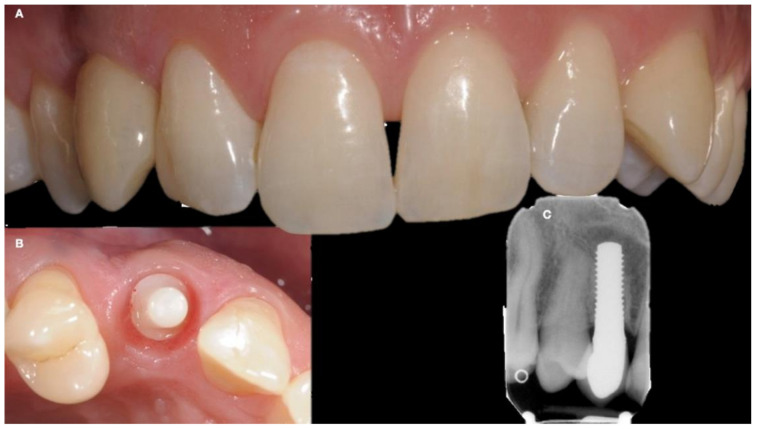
Definitive crown cementation (A,B); An endoral X-ray confirms the ideal integration of the implant (C).
